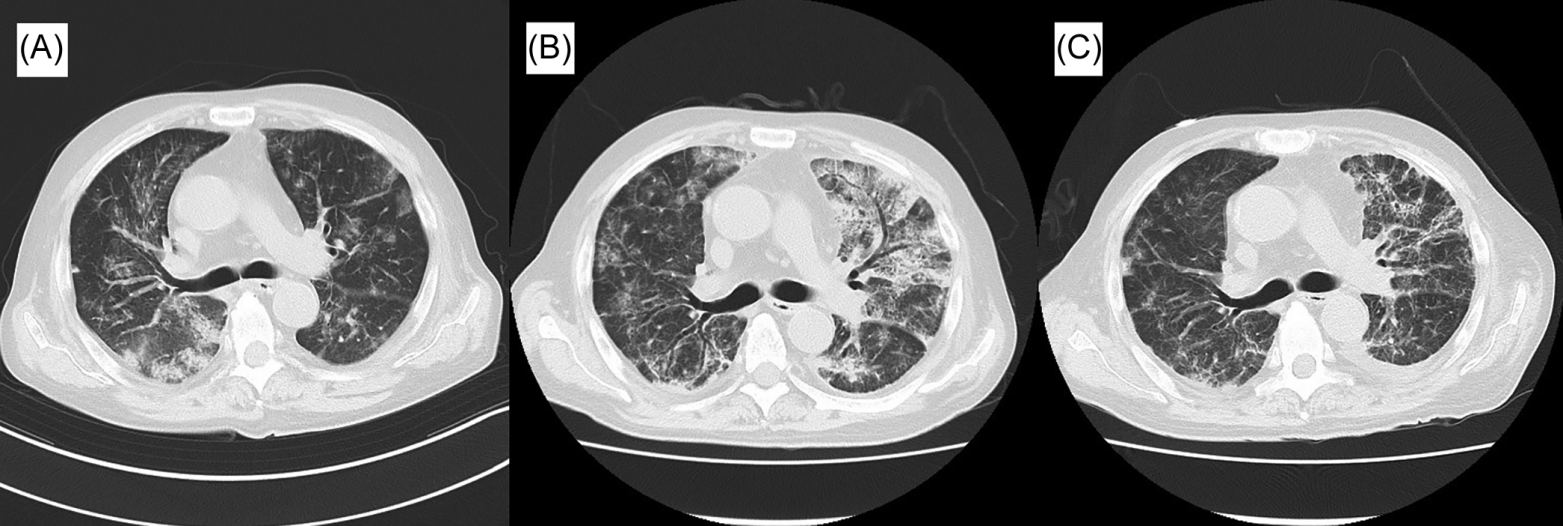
Progression of chest computed tomography (CT) imaging findings. (A) Chest CT scan obtained during hospitalization due to respiratory failure (hospital day 1). (B) Chest CT performed when the pulmonary involvement worsened during the administration of methylprednisolone at a dose of 40 mg/day after the initial methylprednisolone pulse therapy (hospitalization day 11). (C) Chest CT performed when the pulmonary involvement worsened during the administration of methylprednisolone at a dose of 80 mg/day after the second methylprednisolone pulse therapy (hospitalization day 18).
